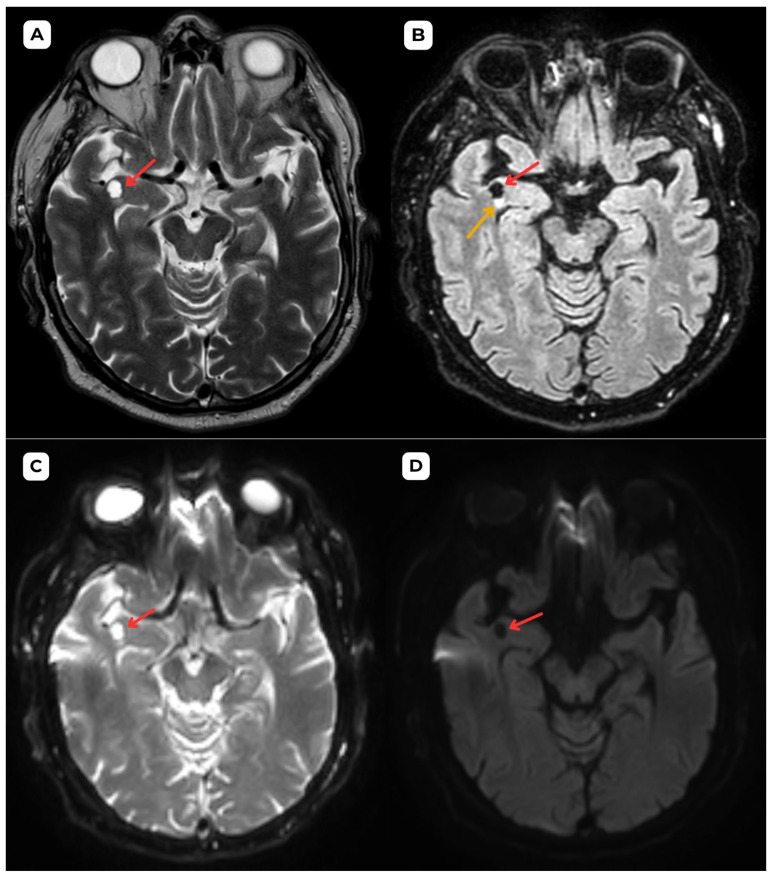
Accompanying second patient: Brain MRI of a 67-year-old male. (A) Axial T2-weighted sequence. A cystic lesion (red arrow) located in the upper–middle frontal gyrus, anterior part of the right temporal lobe, measuring 0.8 × 0.7 cm and up to 1.25 cm AP, accompanied by very minimal edema or gliosis, in contrast with the MRI findings in the first patient case. (B) The axial T2-FLAIR sequence shows the lesion in the same location with better visualization of the CSF contents and emphasizes the very minimal hyperintense perifocal gliosis or edema (yellow arrow). (C) The ADC map displays a high value and no diffusion restriction on (D) DWI. Similarly to the first patient, these changes show no signs of potential malignancy. Red arrow—opercular (type IV) perivascular space; yellow arrow—surrounding edema. In both of these cases, MRI images were acquired with the Siemens “MAGNETOM Sola” 1.5T system. In both cases, the cerebrospinal fluid signal is visible in all MRI sequences. In MRI imaging, opercular perivascular spaces can also be differentiated based on their appearance: round, oval, or tubular, well-defined cystic structures that do not contain calcifications, hemorrhagic elements, or high-protein content structures. In both cases, opercular perivascular spaces appear round (Figure 1, Figure 2, Figure 3, Figure 4, Figure 5 and Figure 6). These formations do not enhance with contrast, which also helps to distinguish them from other pathologies. In both of the cases, no contrast enhancement was seen (Figure 1, Figure 2, Figure 3, Figure 4, Figure 5 and Figure 6). It is important to highlight the pathognomonic feature—visualization of the perforating central artery of the perivascular space—using TOF combined with 3D CISS sequences [8].
